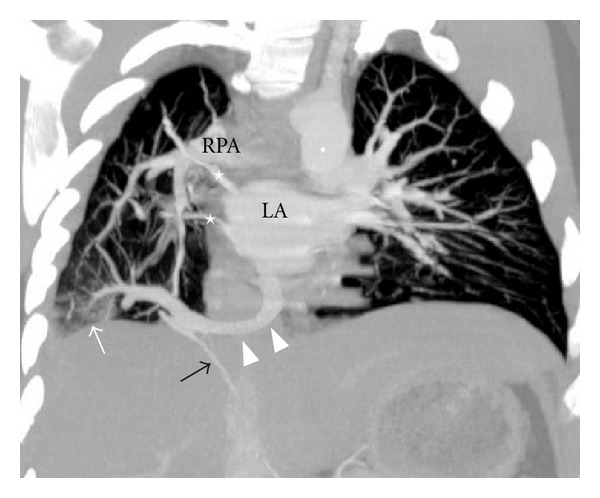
CT angiography maximum intensity projection image in coronal plane depicts right superior and middle pulmonary vein (asterix). Sequestration (white arrow), meandering right inferior pulmonary vein (arrow heads). Anomalous systemic arterial supply (black arrow). Left atrium (LA). Right pulmonary artery (RPA).
